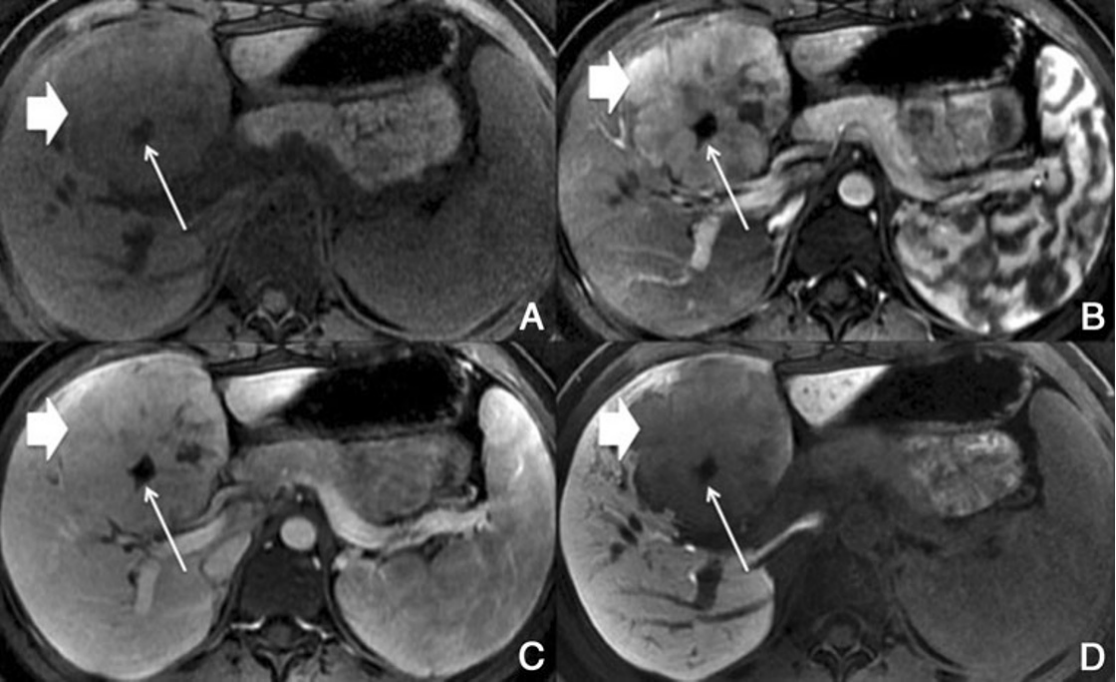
Fibrolamellar-HCC in a 34-year-old man without liver disease. a Pre-contrast MRI, b arterial phase, c PV phase, d HCP. Big nodular lesion in the left lobe isointense in pre-contrast imaging, with arterial enhancement, washout in the PV phase and no uptake of Gd-EOB-DTPA in the HCP (*thick arrow*). The central scar (*thin arrow*) remains hypointense in all sequences
